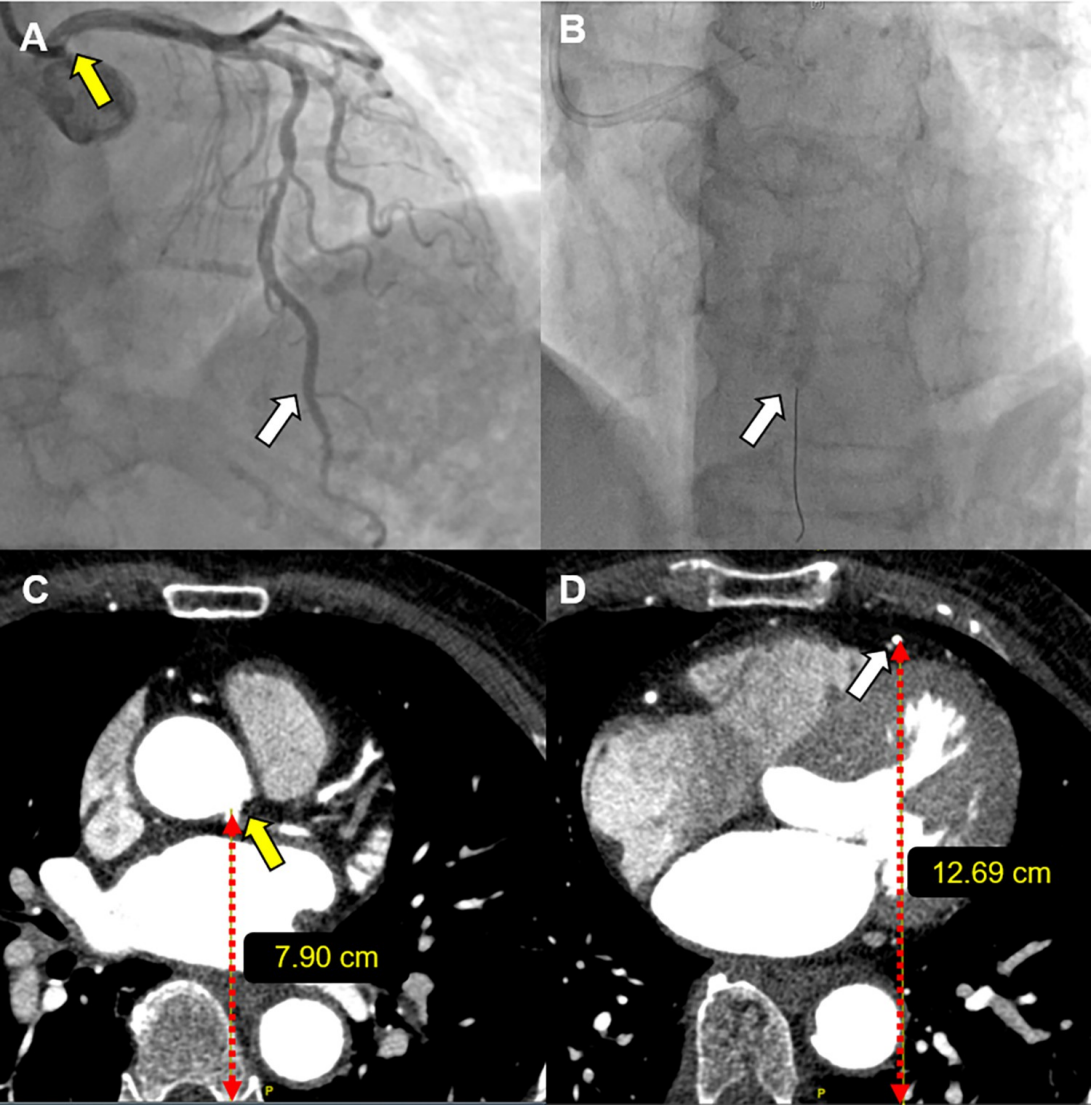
Measurement of aortocoronary height difference. Representative case of an 80-year-old woman with typical angina. Coronary angiography showed 50% stenosis in the mid left anterior descending artery on the anterior-posterior-cranial view (A). The pressure wire was placed as distal as possible. A small diagonal branch next to the pressure sensor was the landmark for heightening the wire (white arrow) (B). The height was measured from the base of the computed tomography (CT) image to the left main ostium (yellow arrow) (C). Then, the landmark (small diagonal branch) was traced, and the height was measured from the base of the CT image (D). The difference between the two measurements can be used to estimate the height from the ostium to the pressure sensor. CT, computed tomography.
